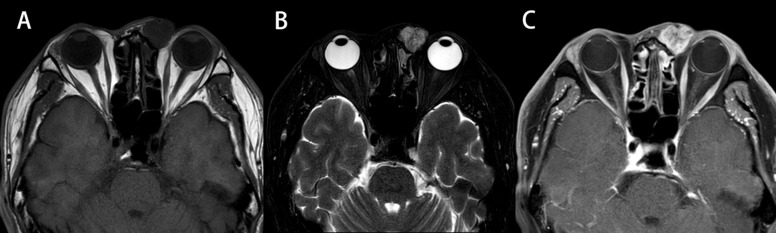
Magnetic resonance imaging of patient 2. Circular masses can be seen in the inner canthus. The intensity of the area in T2-weighted imaging (B) is higher than that in T1-weighted imaging (A), and the signals in the area are uneven. After enhancement (C), the enhancement was uneven and obvious. Shallow lobules can be seen at the margin. The lesions involved the lacrimal region and the superior nasolacrimal duct. Bone discontinuity in the right orbital wall. Bilateral frontal sinus, ethmoid sinus and maxillary sinus mucosa thickened.
